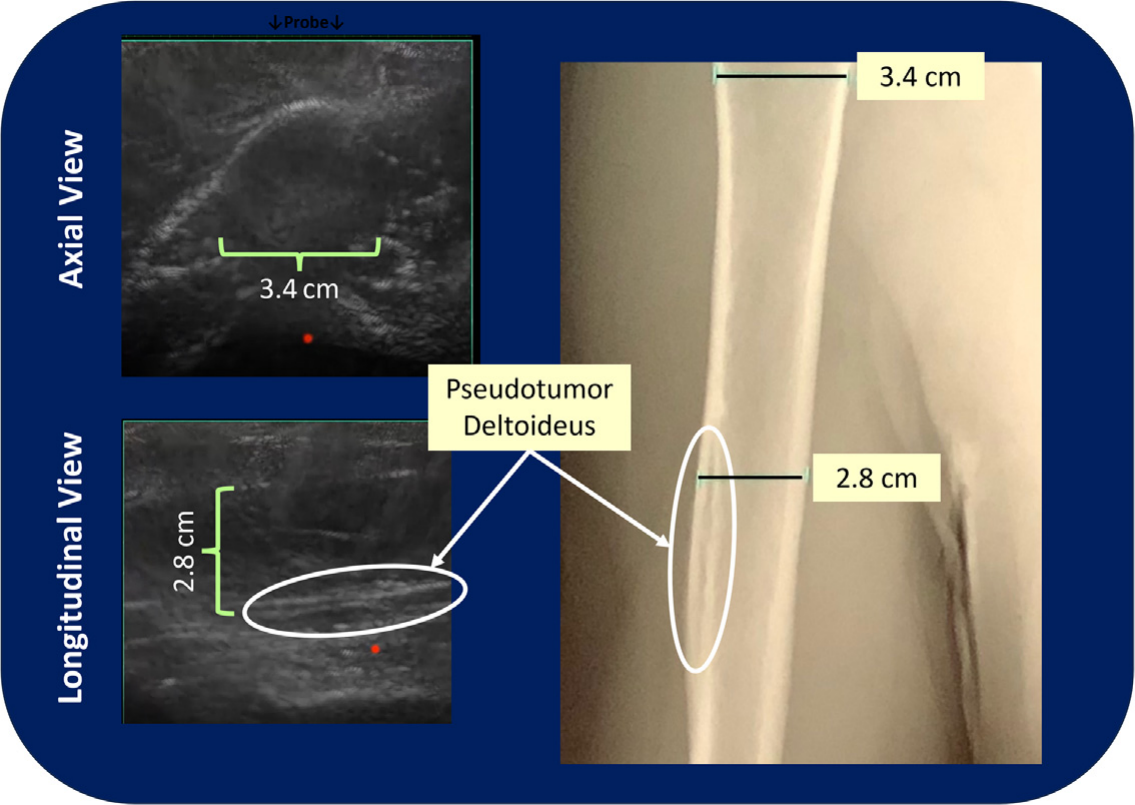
Comparison of long bone images obtained using computer echo tomography (CET) and standard x-ray. To demonstrate the anatomic fidelity of CET, images of the right humerus of an adult male volunteer were obtained using both standard x-ray and the K3900. The measurements obtained were generated by the software of the 2 systems with an experienced radiologist marking the distances to be measured. Serendipitously, the volunteer was found to have a normal anatomic variant known as *pseudotumor deltoideus,* which is seen as a cortical lucency below the insertion of the deltoid muscle on x-ray. Identification of this unusual finding using CET imaging suggests that visualization of nondisplaced fractures of long bones may also be possible.
